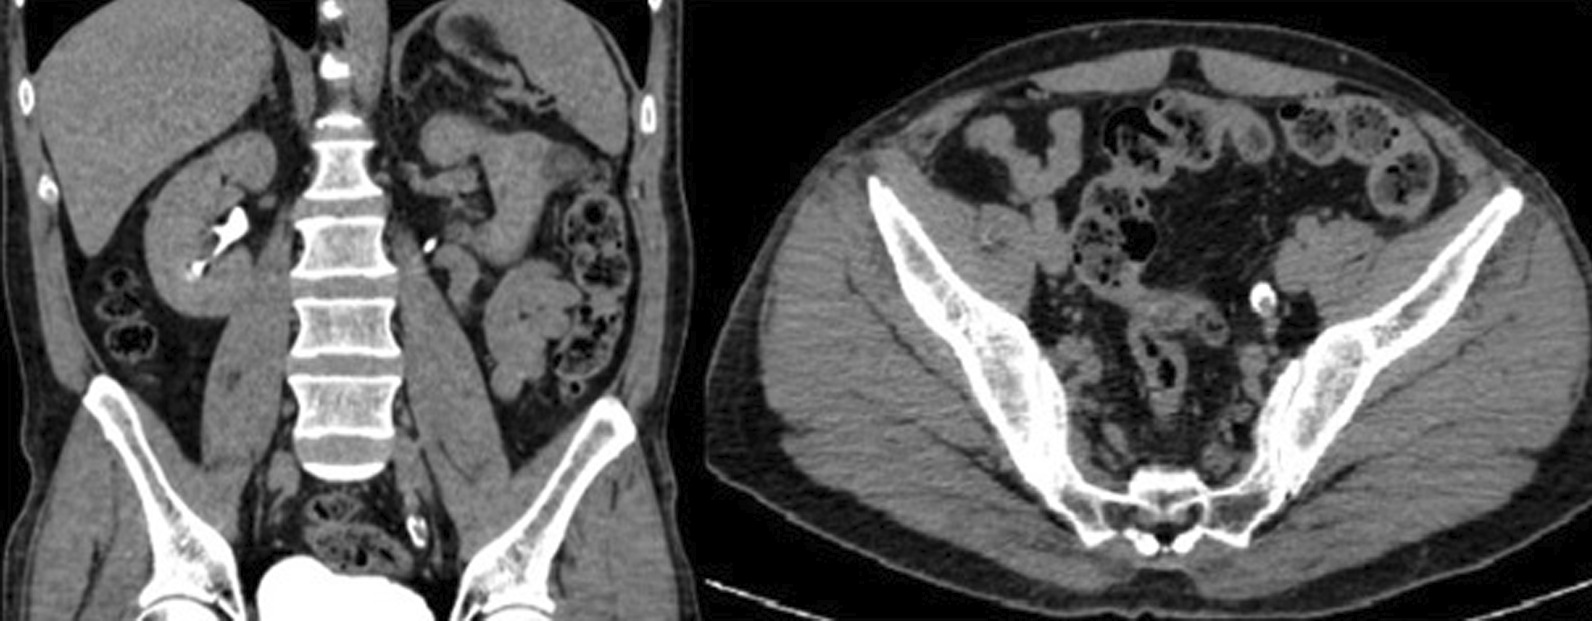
Filling defect in the urinary system in the excretory phase of triphasic dynamic abdominal computed tomography; coronal and transverse plane images of fibroepithelial polyps located in the left ureter
